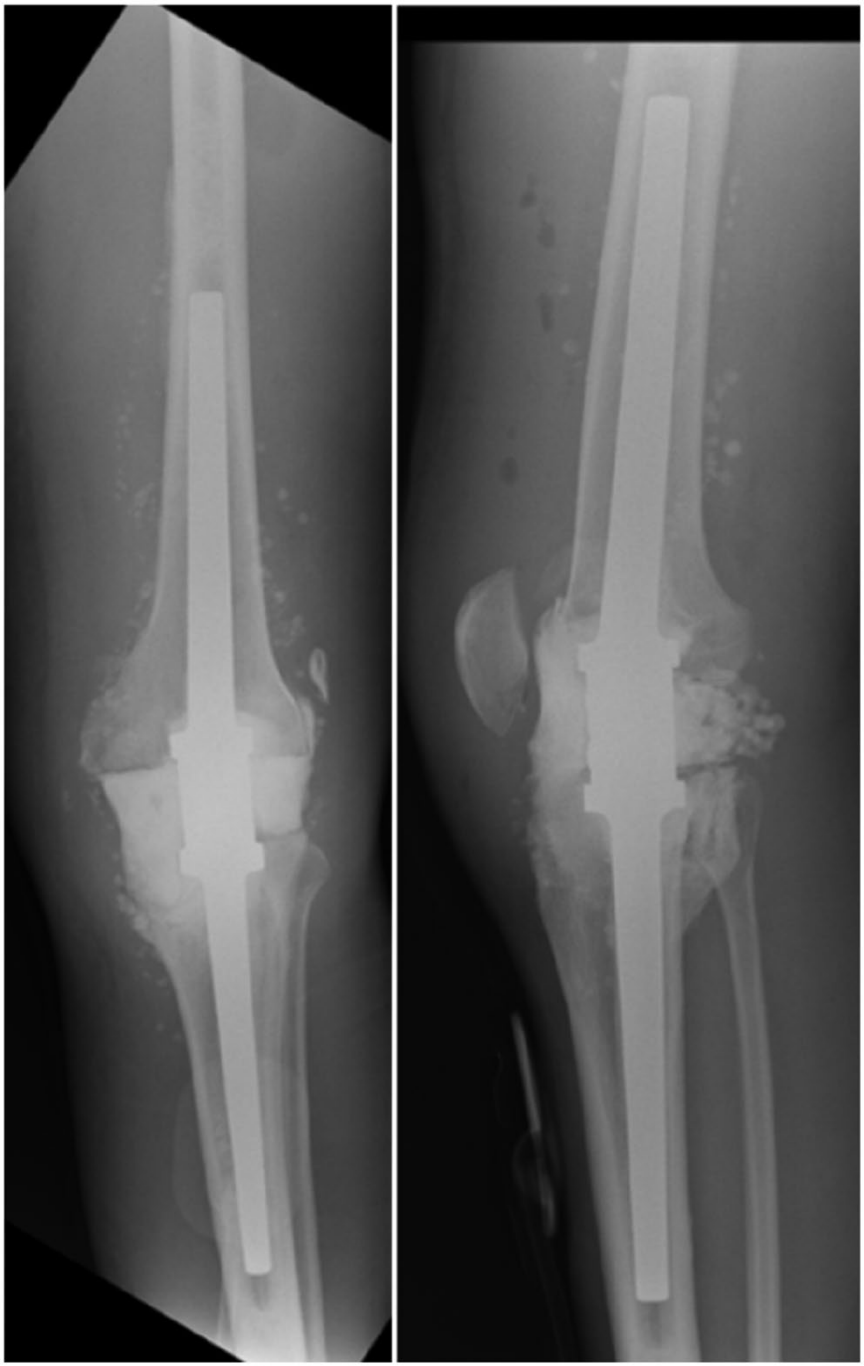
Radiograph images in two planes of a static spacer constructed from arthrodesis nail
